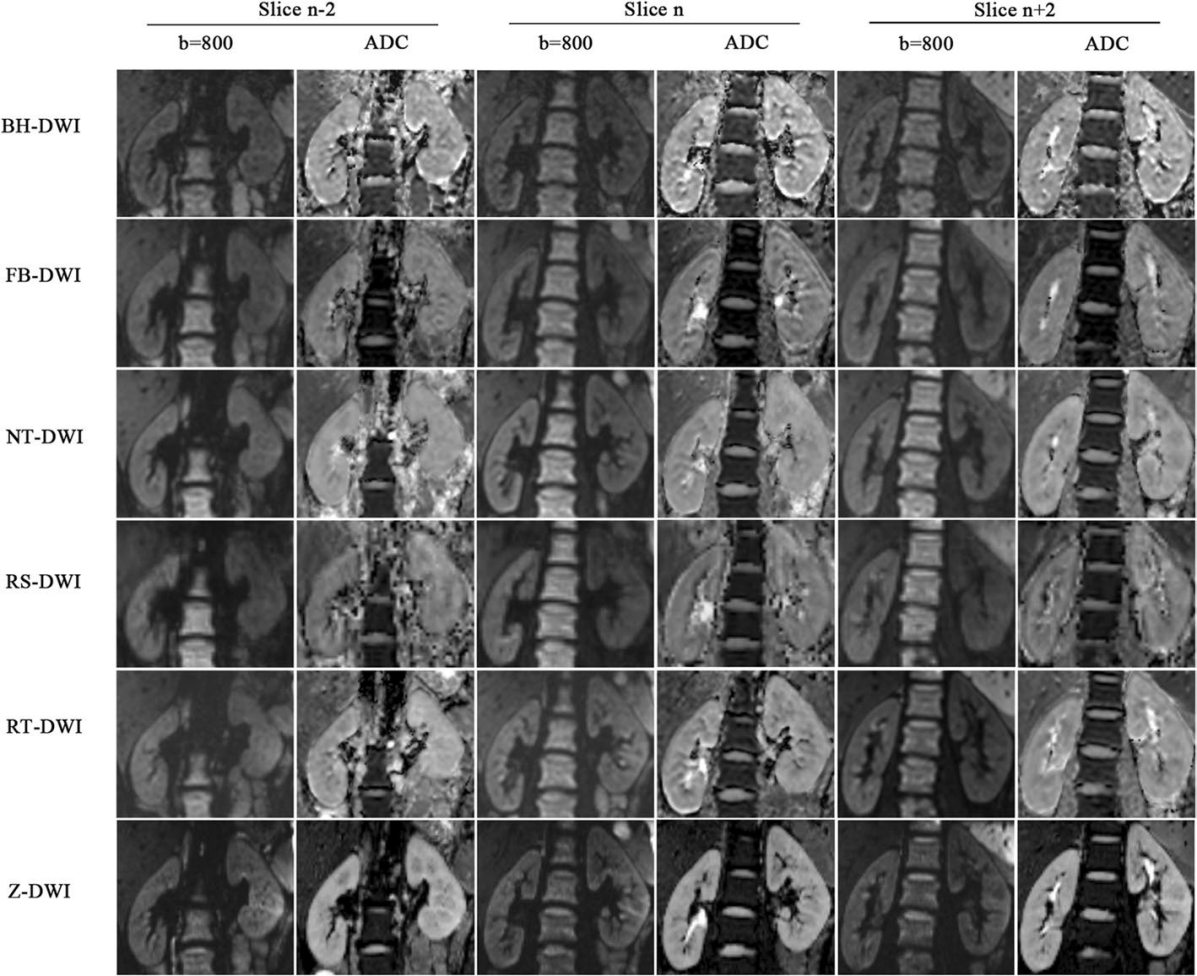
Comparisons of image quality of BH-DWI, FB-DWI, NT-DWI, RT-DWI, RS-DWI and Z-DWI at b = 800 s/mm^2^ and corresponding ADC map. The image quality of Z-DWI was significantly different from BH-DWI, FB-DWI and RS-DWI in the three representative section (n-2 slice, n slice and n + 2 slice) in clarity of the renal cortex and medulla (ADC map) (all *P* < 0.05). Z- DWI was slightly superior to RT- DWI and NT-DWI in the three representative section (n-2 slice, n slice and n + 2 slice) in sharpness of boundaries (ADC map); however, the difference in image quality between the three was also not significant (all *P* > 0.05).
